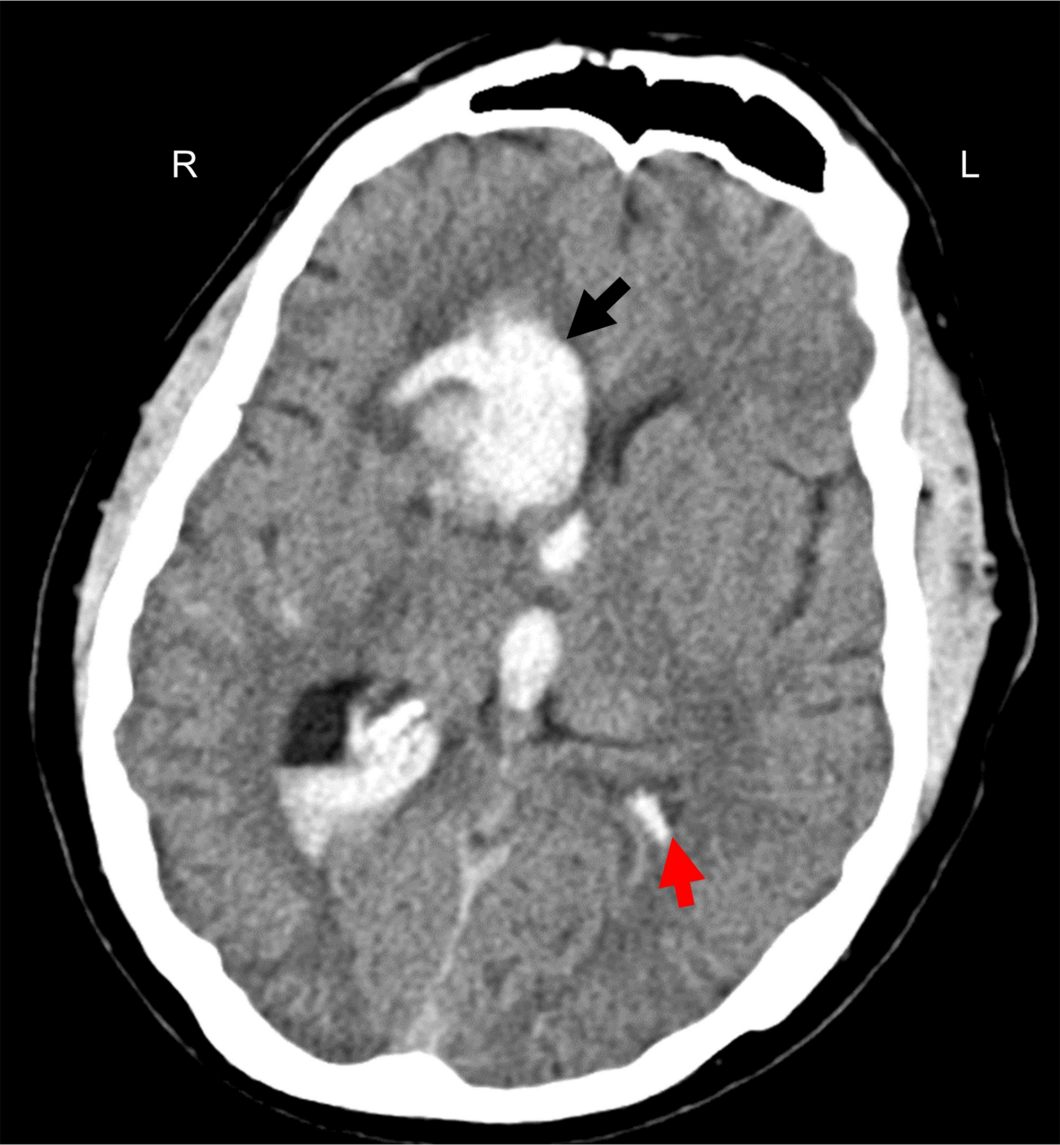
Repeat computed tomography (CT) head six hours (following morning) after presentation. Increased size of the intracerebral hemorrhage (23 cm^3^), a collapsed left lateral ventricle (red arrow) secondary to cerebrospinal fluid drainage, entrapment of the left temporal horn, and peri-hematoma edema with 6.5 mm of midline shift.
